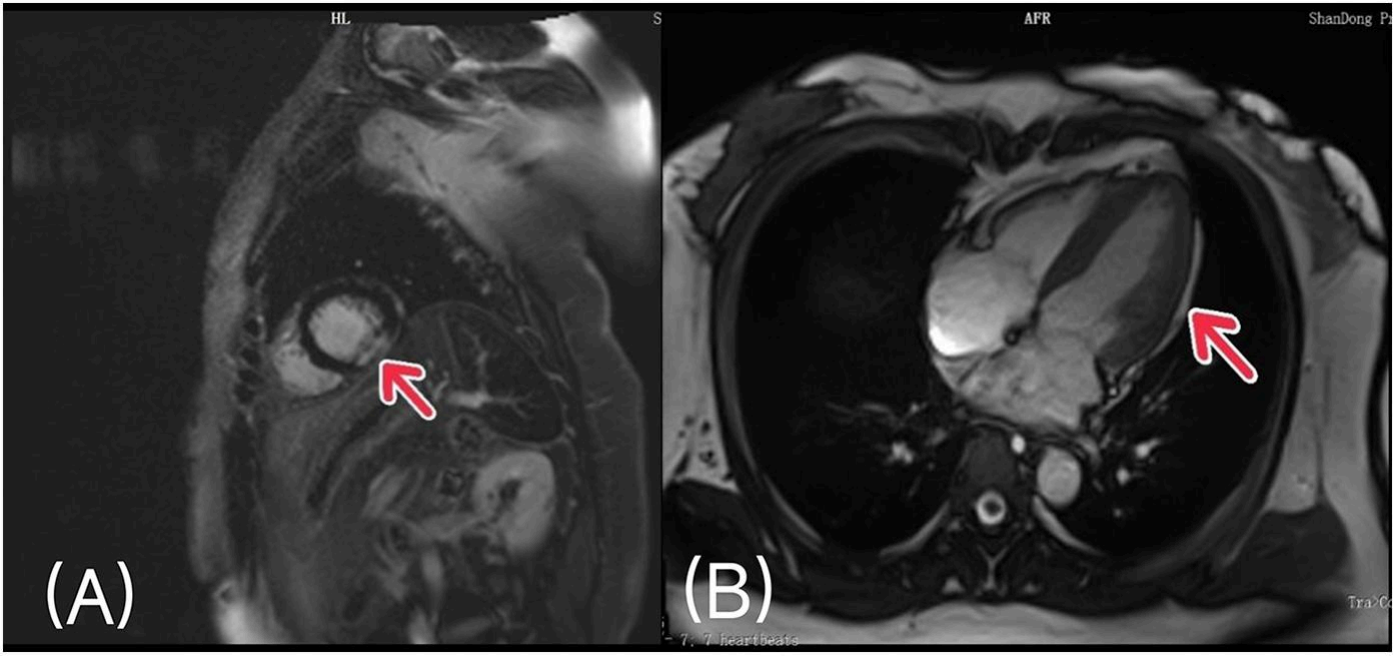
Cardiac magnetic resonance imaging: (A) patchy perfusion defects and delayed enhancement in the inferior wall of the middle and apical segments of the left ventricle. (B) The left ventricular free wall had increased muscle trabeculae and incomplete myocardial compaction.
